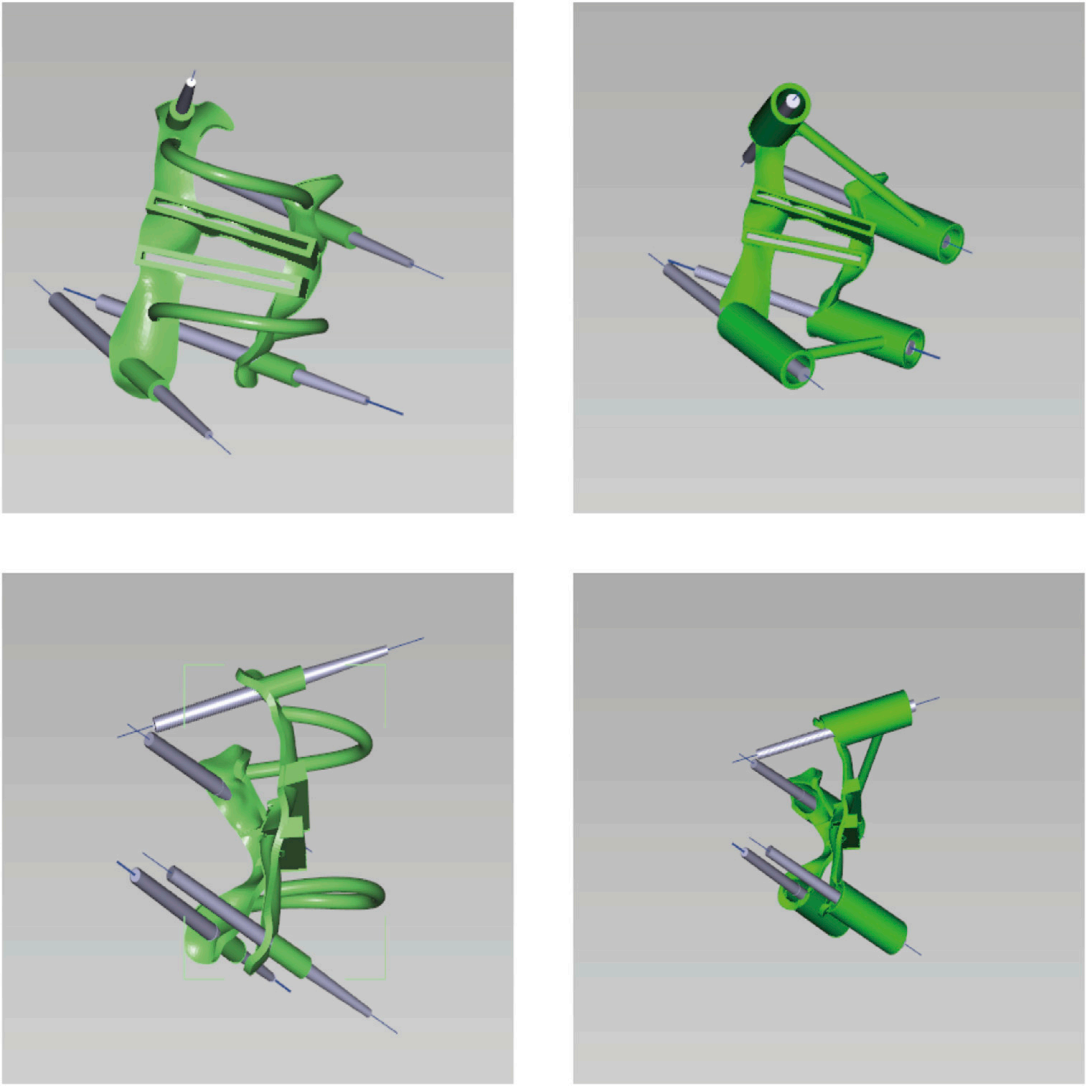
Anteroposterior and lateral views of the osteotomy guide. The width of the cutting notch was set to 1 mm, adjusted according to the specifications of the ultrasonic osteotome.
